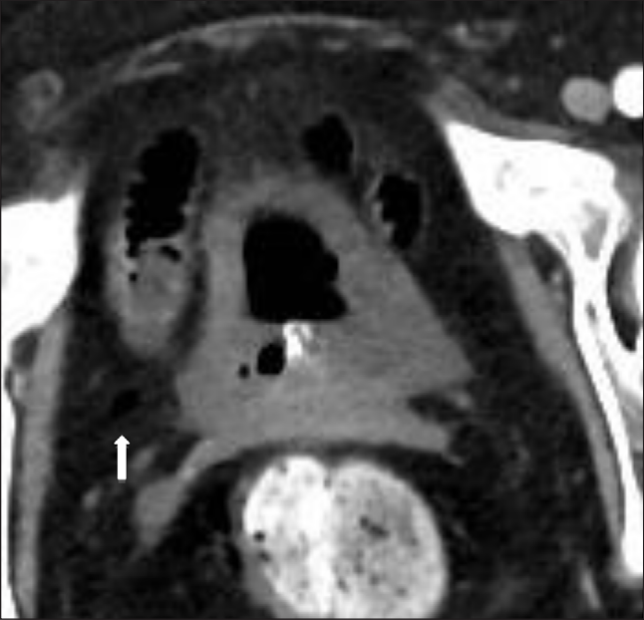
One-week later follow-up CT was performed, this showed a spontaneous healing of the bladder wall with only a small residual focus of free air in the Retzius space on the right (white arrow).
